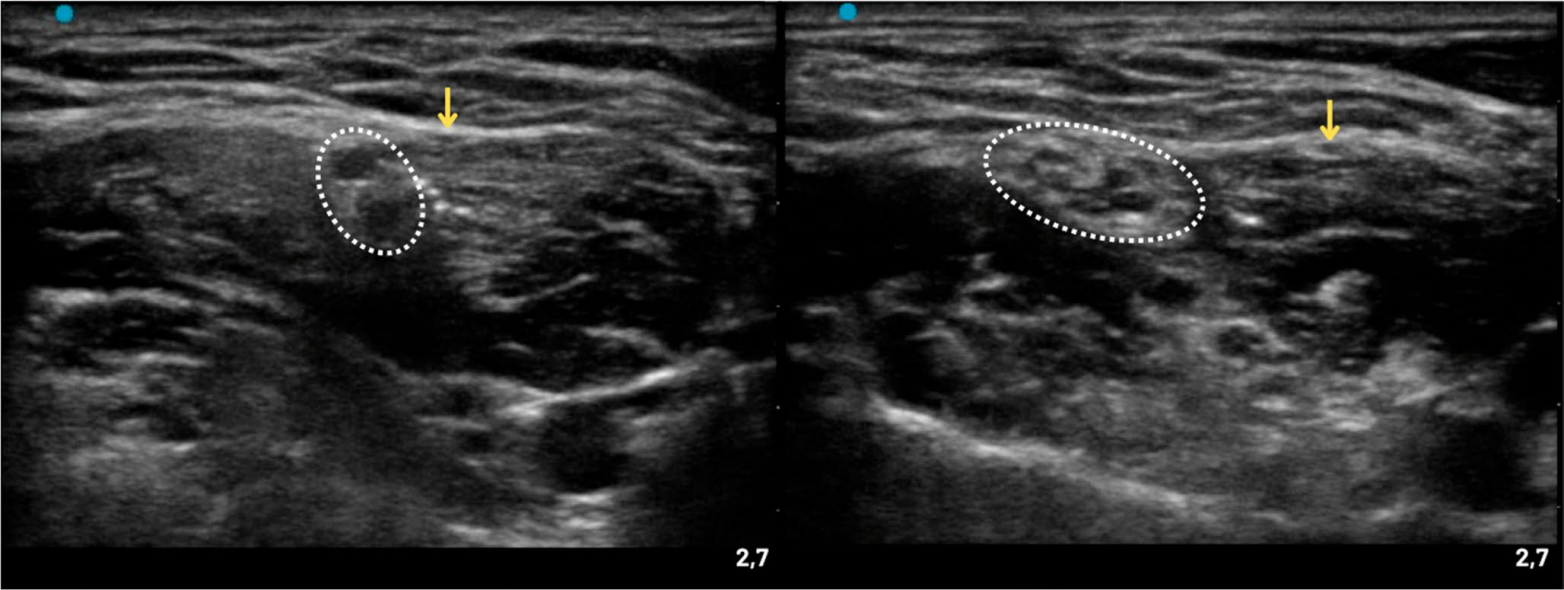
Ultrasound images comparing interscalene brachial plexus (left) and superior trunk (right). Neural structures are highlighted by white dotted circles. The yellow arrow indicates the relationship with the phrenic nerve. In the interscalene view, the plexus lies in close proximity to the phrenic nerve, whereas the superior trunk is located at a greater distance, providing a larger safety margin and a lower likelihood of phrenic nerve involvement
